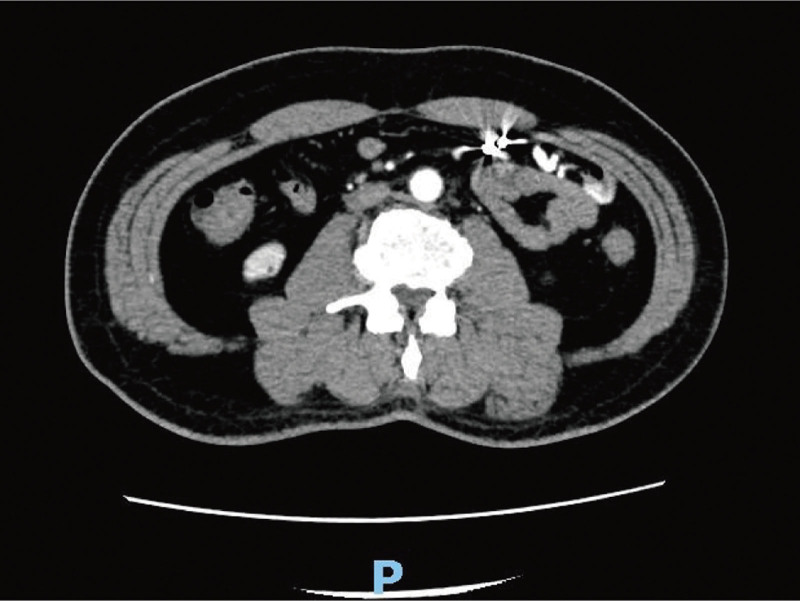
Vascular malformation of the small intestine in the left upper quadrant was shown by abdominal contrast-enhanced computed tomography (CT).
